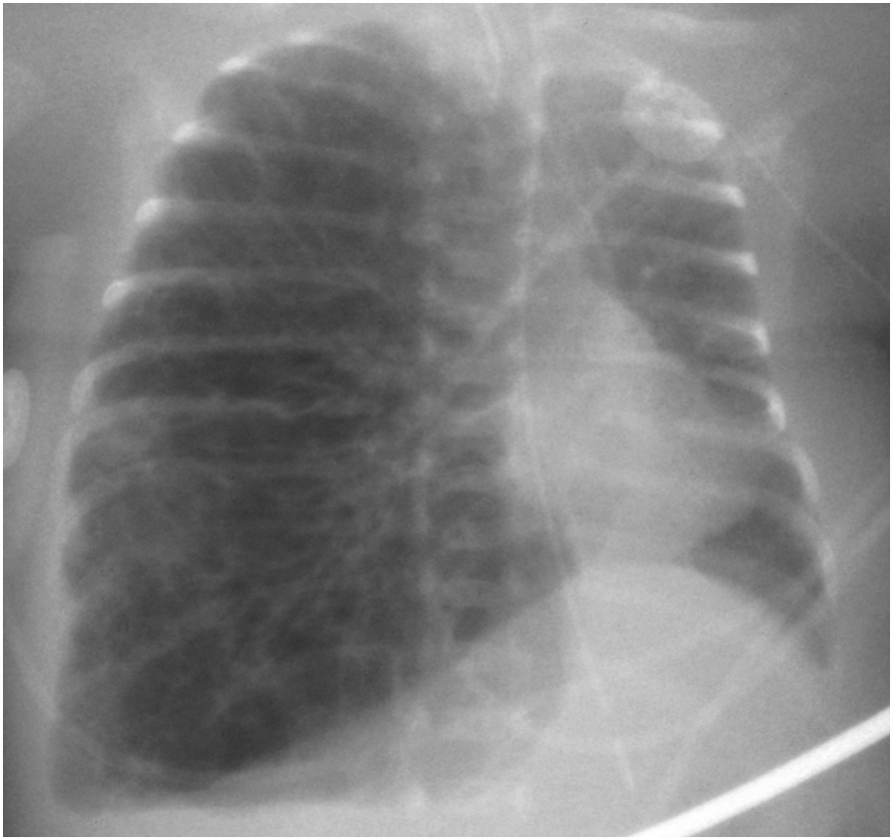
A chest X-ray obtained before lung puncture. The chest radiograph obtained just before decompression shows diffuse pulmonary interstitial emphysema in the right lung and a mediastinal shift to the left.
